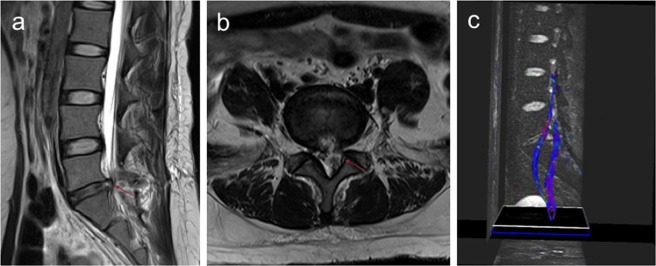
Lumbar disc MRI and lumbosacral nerve root DTI scan at 3 days post-surgery. (a) is the sagittal position of the patients’ MRI 3 days post-surgery, and the herniation has disappeared, leaving the posterior longitudinal ligament with the “grape skin” phenomenon. (b) is the axial position of the patient’s MRI 3 days post-surgery, adequate spinal canal decompression can be observed. (c) is the nerve root reconstructed by DTI 3 days post-surgery, no obvious recovery of the compressed nerve microstructure can be observed.
